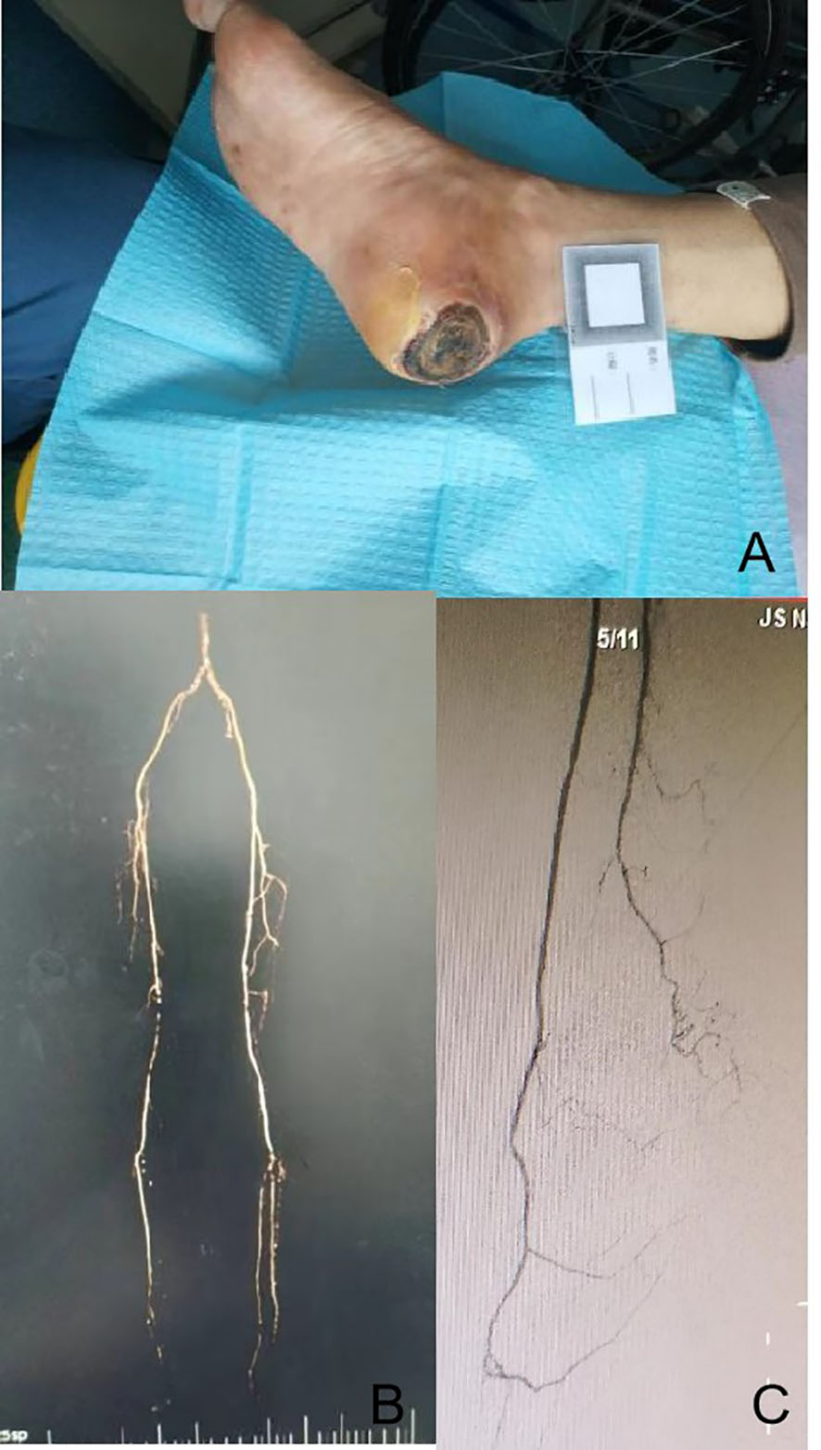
Physical examination of the wound, preoperative CTA examination of lower limb vessels, and angiography after lower limb arterial reconstruction. It was a round ulcer of approximately 5 cm × 5 cm in diameter, which was covered with large black scabs, and the tissue around it was cool, dark red, and swollen (A). Long segment occlusion of the inferior segment of the right superficial femoral artery with segmentary severe stenosis (B). The vascular lumen of the superficial femoral artery, popliteal artery, anterior tibial artery, peroneal artery, and some arterial arches of the foot were unobstructed in the affected limb after lower limb arterial reconstruction (C). CTA, computed tomography angiography.
